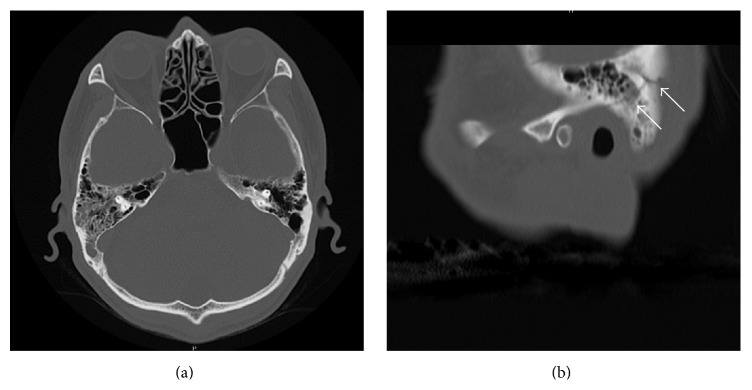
CT scan of the head without contrast 12 days after trauma: (a) axial cut (bone window) cannot show obvious fractures; (b) sagittal cut of the reconstructed CT demonstrates a fracture line in the right temporal bone (arrow).
